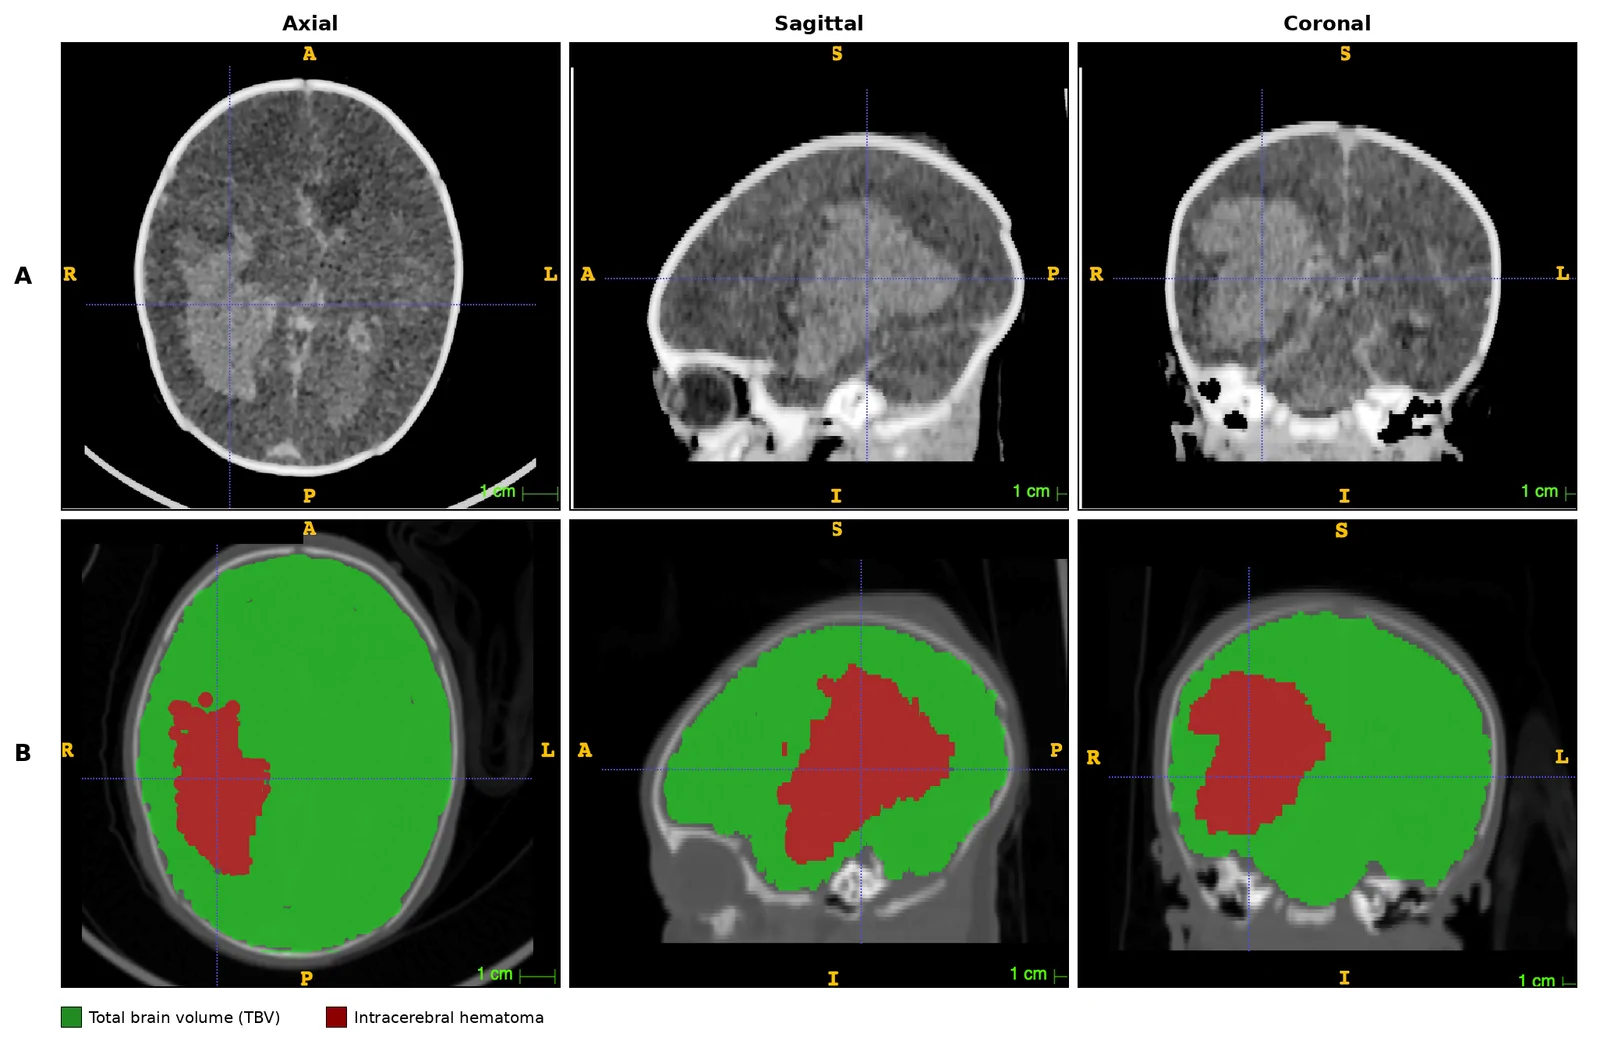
Quantitative neuroimaging analysis using ITK-SNAP. (A) Baseline non-contrast head CT in axial, sagittal, and coronal planes, showing a spontaneous pediatric intracerebral hemorrhage with mass effect. (B) Corresponding three-dimensional volumetric segmentation, with total brain volume (green) and intracerebral hematoma (red) labeled to compute hematoma-to-brain volume ratios and midline shift. Orientation labels follow standard radiological convention: A, anterior; P, posterior; S, superior; I, inferior; R, right; L, left.
